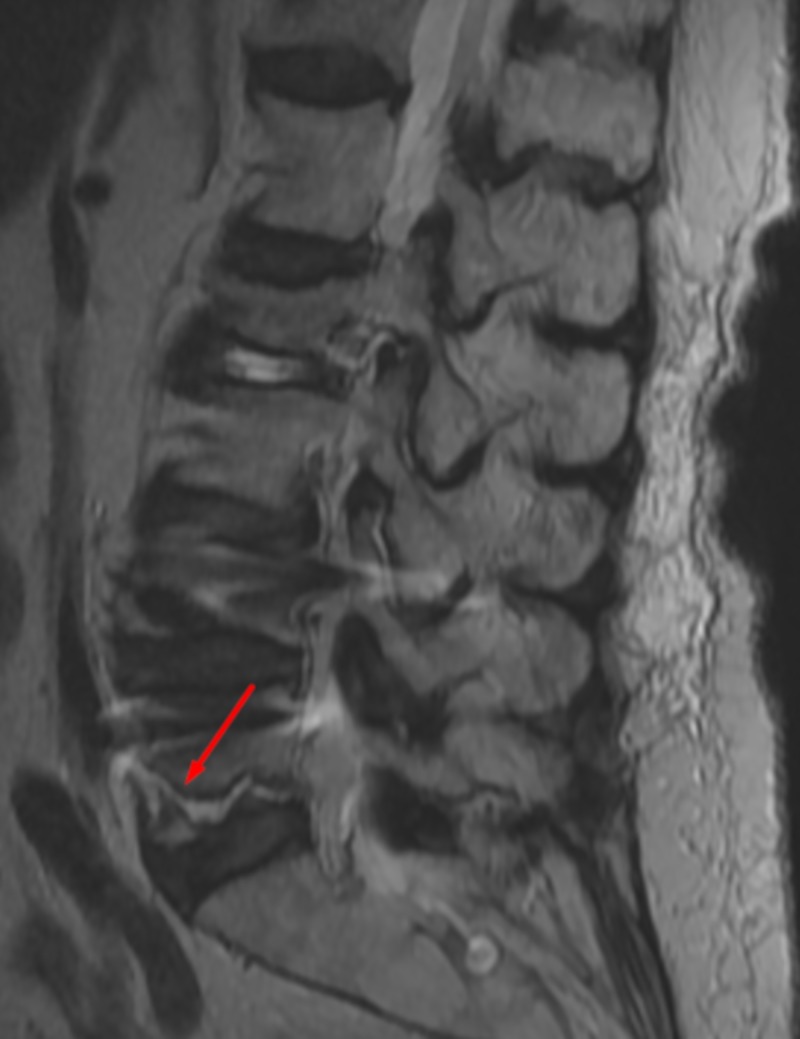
Sagittal Lumbar MRI Sagittal lumbar magnetic resonance imaging (MRI) displaying fracture with associated edema on L5. Arrow indicates fracture.
